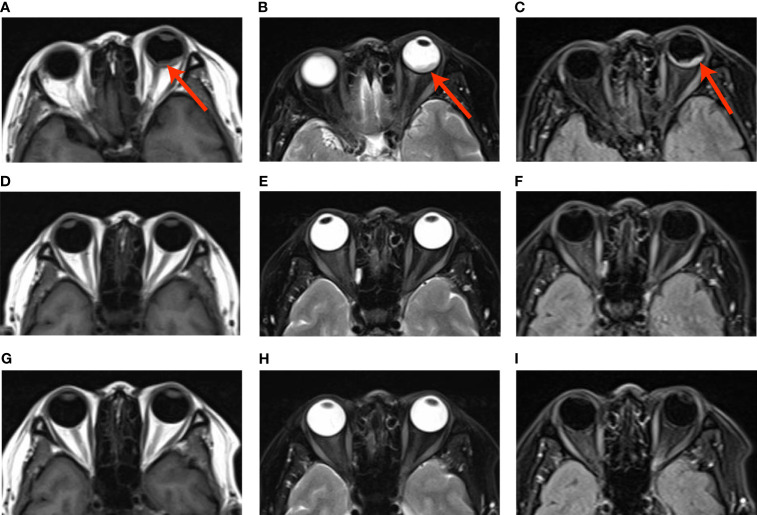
(A–C) Basal MRI-scan of the eyes, (D–F) reduction of the abnormal tissue in the left eye in 6th month crizotinib theraphy; (G–H). No progression of malignancy on the 14th month crizitinib therapy.
